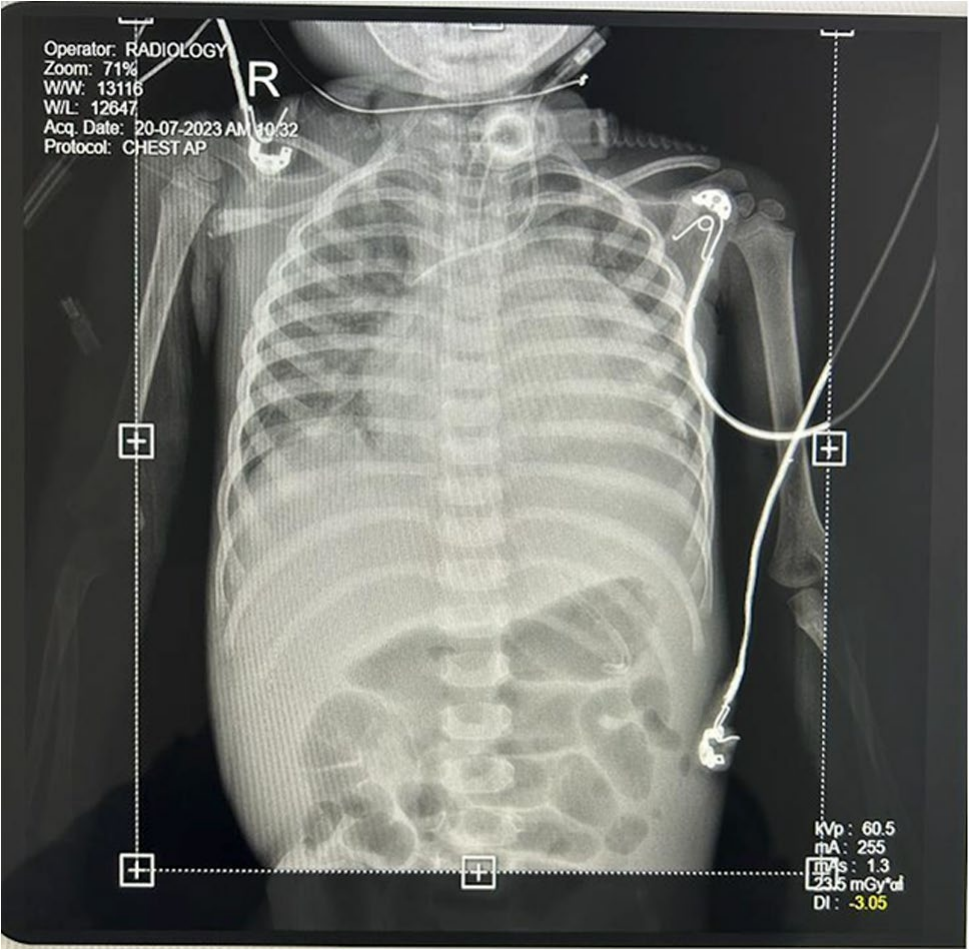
Anterior–posterior (AP) chest X-ray of the patient with dilated cardiomyopathy (DCMP)
